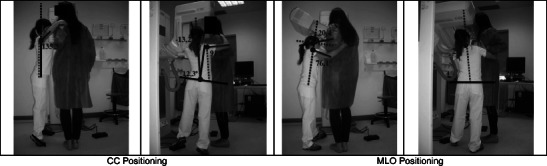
Postures assumed by a radiographer smaller than the patient
